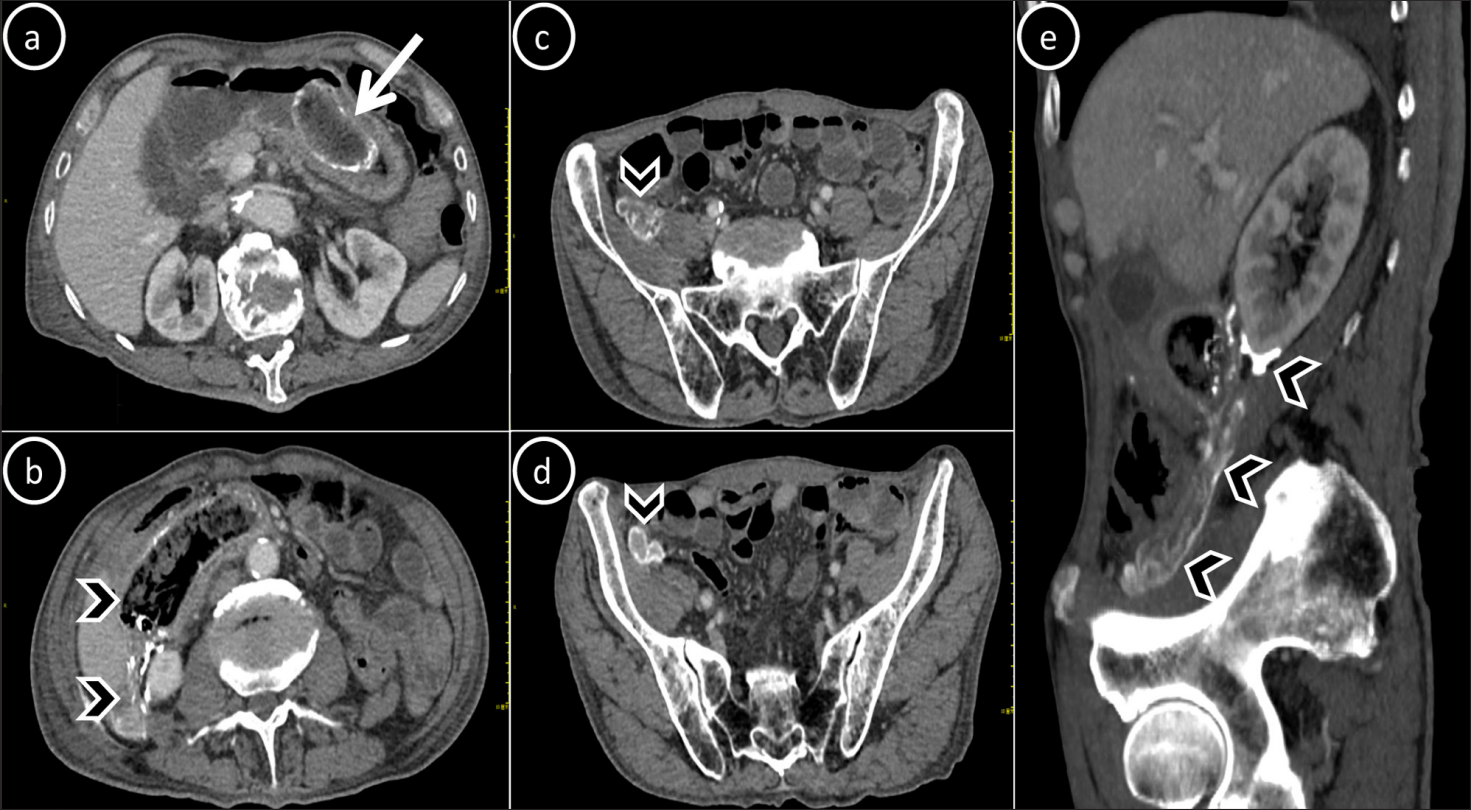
Axial (a–d) and sagittal reconstructed (e) contrast-enhanced abdominal CT scan of an 81-year-old patient with gastrointestinal (arrow) and peritoneal (arrowheads) barium granulomas.
